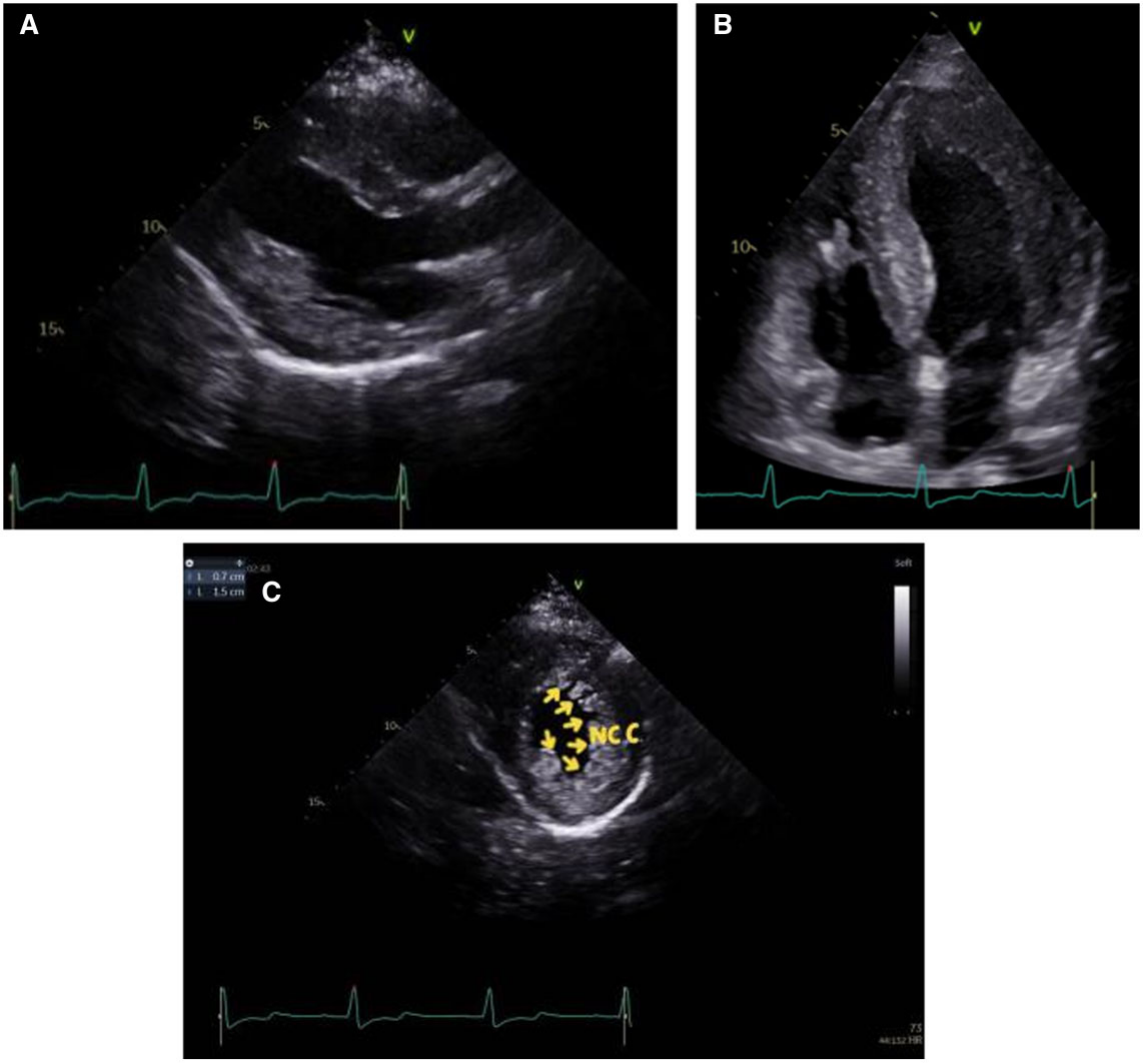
Two-dimensional echocardiography of patient 2. Arrows indicate non-compacted myocardium (NC). C indicates compacted myocardium.
